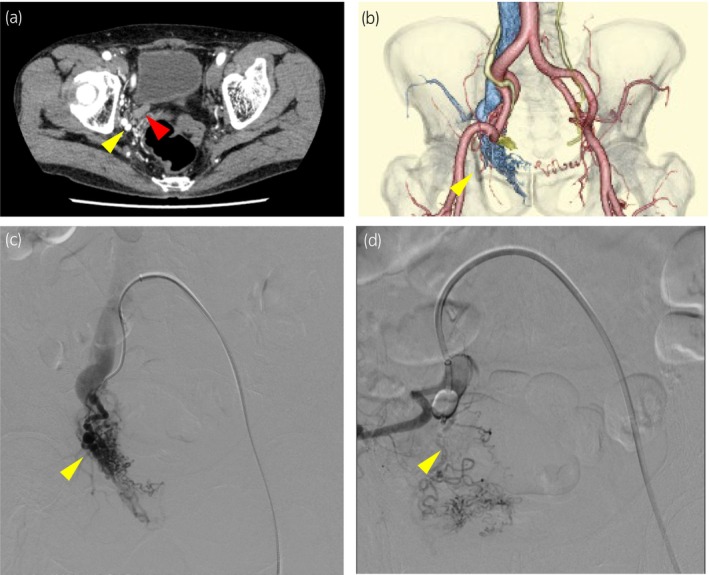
(a) and (b) Shows that enhanced CT reveals right ureteral tumor with right pelvic AVM near the right lower ureter. Arterial‐phase right internal iliac angiography shows numerous expanded arteries in the AVM (c). After inflating the occluding balloon, angiography confirms correct placement of the balloon in the right internal iliac artery and reveals stagnant flow (d). Red arrowhead shows the urethral tumor and yellow arrow shows the AVM.
